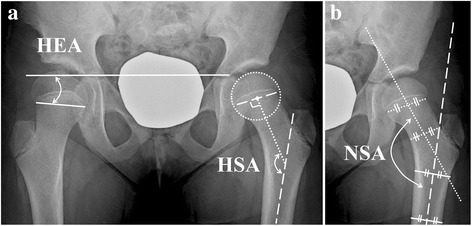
a Hilgenreiner-epiphyseal angle is the angle between the Hilgenreiner line and a line connecting the medial and lateral end of the proximal femoral physis on the hip anteroposterior radiograph. Head-shaft angle is the angle between the proximal femoral shaft axis and a line which is drawn though the center of the proximal femoral epiphysis and perpendicular to the proximal femoral growth plate. Hilgenreiner-epiphyseal angle of the right hip is 6 degrees, and head-shaft angle of the left hip is 152 degrees on this radiograph. b Neck-shaft angle is the angle between the axis of the proximal femoral shaft and the axis of the femoral neck, which links the midpoints of neck diameter at both levels of the subcapital and the base of neck areas. Neck-shaft angle of the left hip is 140.5 degrees in this radiograph
